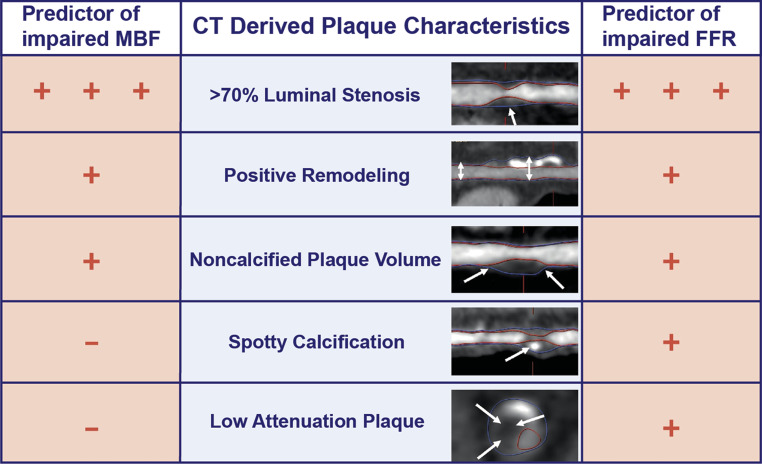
The association of CCTA derived plaque characteristics with impaired hyperaemic MBF measured by [^15^O]H_2_O PET and invasively measured FFR. Driessen et al. studied the effect of CT-derived plaque characteristics on hyperaemic MBF and FFR and demonstrated luminal stenosis severity to be the strongest predictor of impaired hyperaemic MBF and FFR. Positive remodelling and noncalcified plaque volume negatively influenced perfusion and FFR, whereas spotty calcification and low attenuation plaque affected FFR but not hyperaemic MBF. Figure adapted from Driessen et al. [12]. Adapted from and with permission of Elsevier. *MBF* myocardial blood flow, other abbreviations as in Figs. 1 and 2
